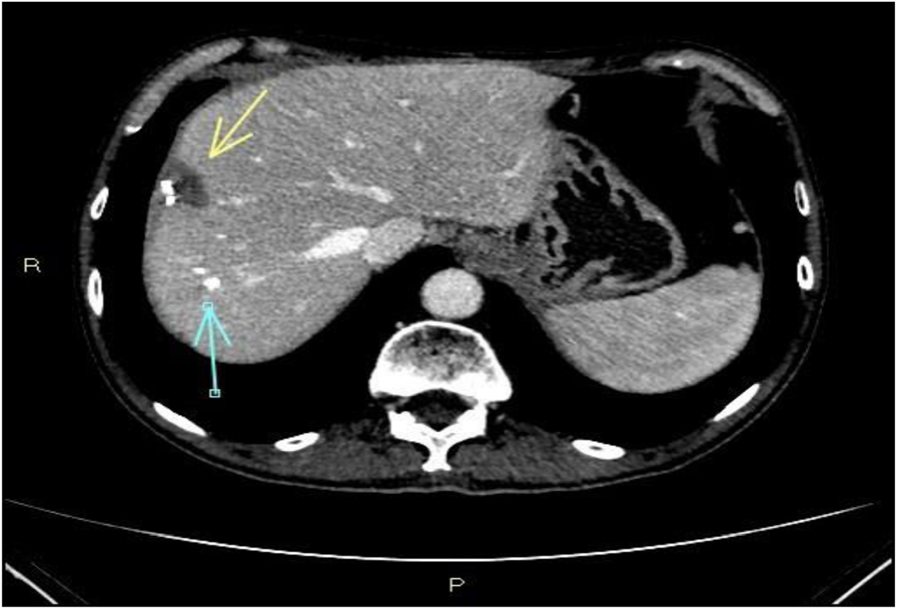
This CT scan image represents the results of the wedge resection and radiofrequency ablation (RFA) of various hepatic lesions. The yellow arrow represents a fluid hepatic collection as a result of radiofrequency. The blue arrow indicates a clip as a result of wedge resection
